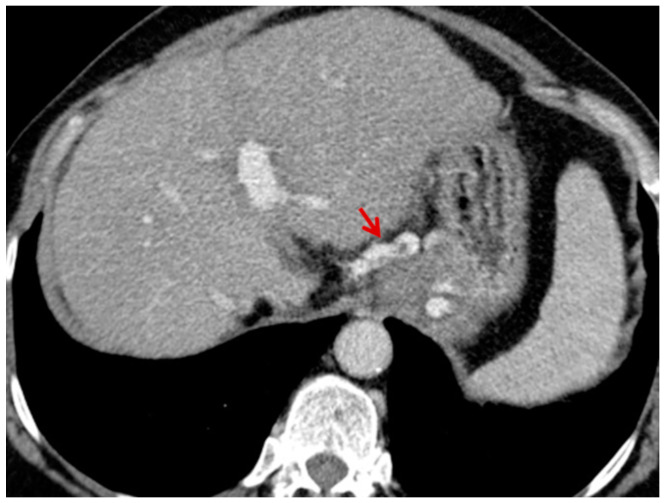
Axial contrast-enhanced CT shows enlarged paragastric varices (red arrow).
